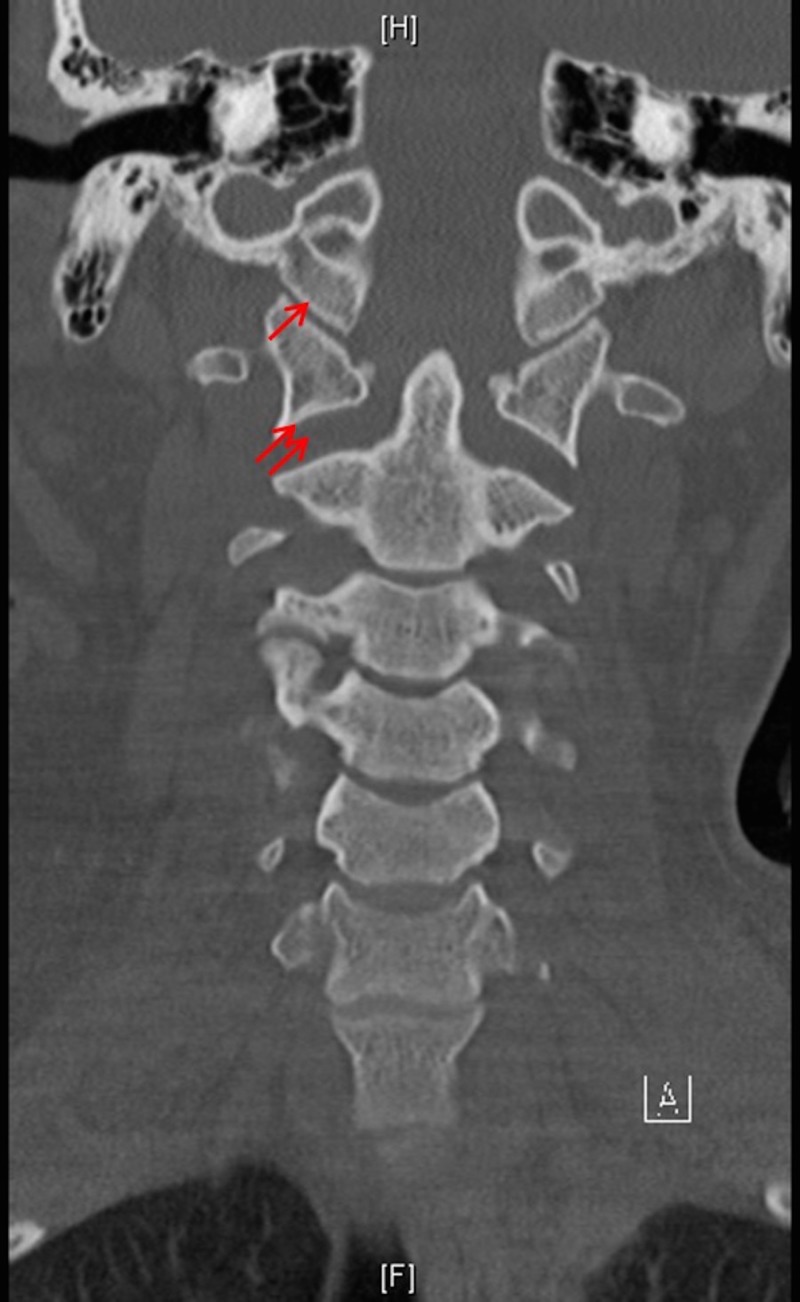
Atlanto-axial joint Coronal reconstructed computed tomography (CT) scan showing normal condyle-C1 interval (CCI) (arrow) and distracted atlanto-axial joints (double arrows).
